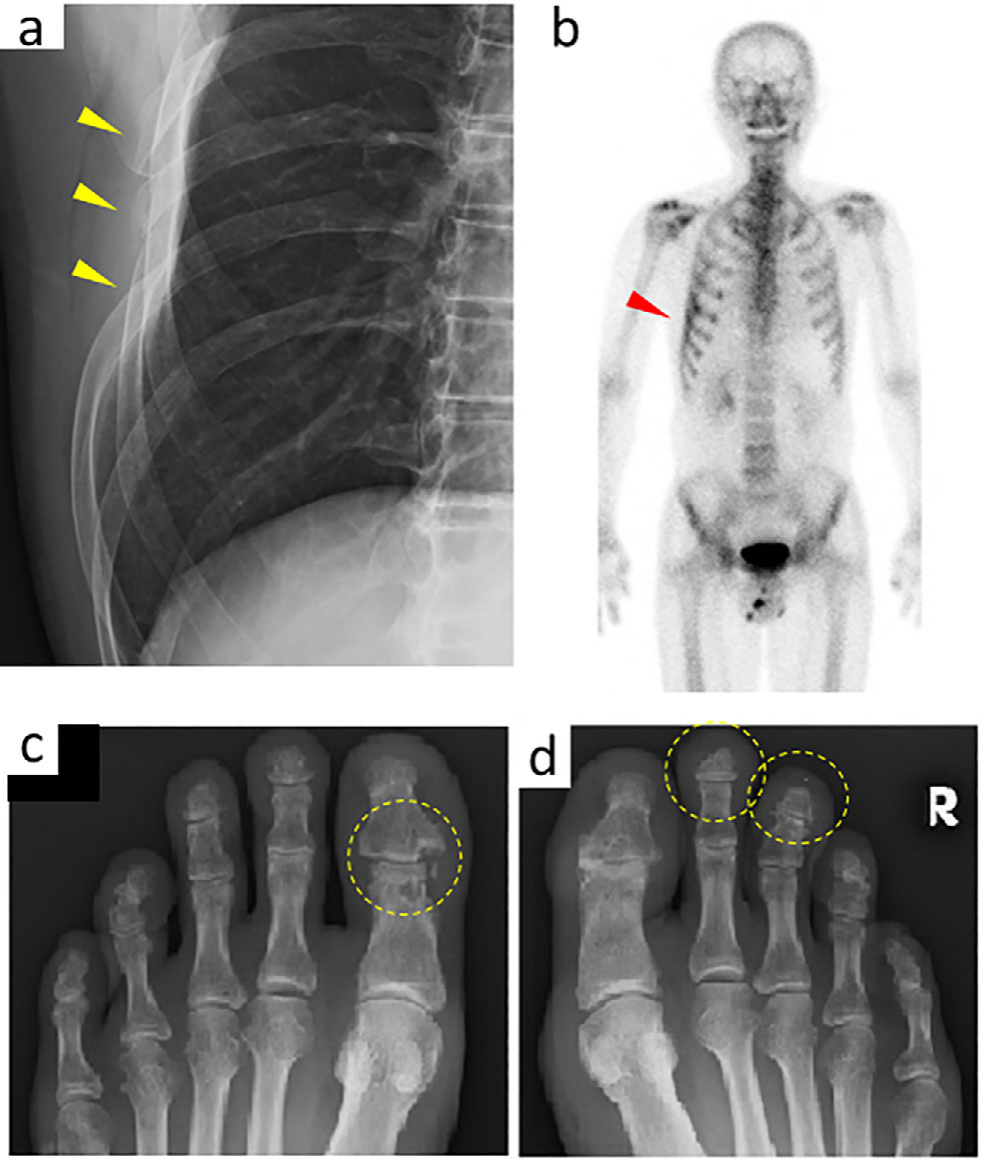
Radiological findings indicate systemic osteolysis in the patient. (*A*) Partial defects in the right ribs (VI–VIII) by a chest radiogram. (*B*) The lateral chest wall on the left side and the thumb of the left hand had hot spots in ^99m^Tc scintigraphy. (*C*) Osteolytic bony defect at the interphalangeal joint of the great toe in the radiogram of the left foot. (*D*) Bony defects on the tips of the second and third toes of the right foot are indicated by dotted circles.
